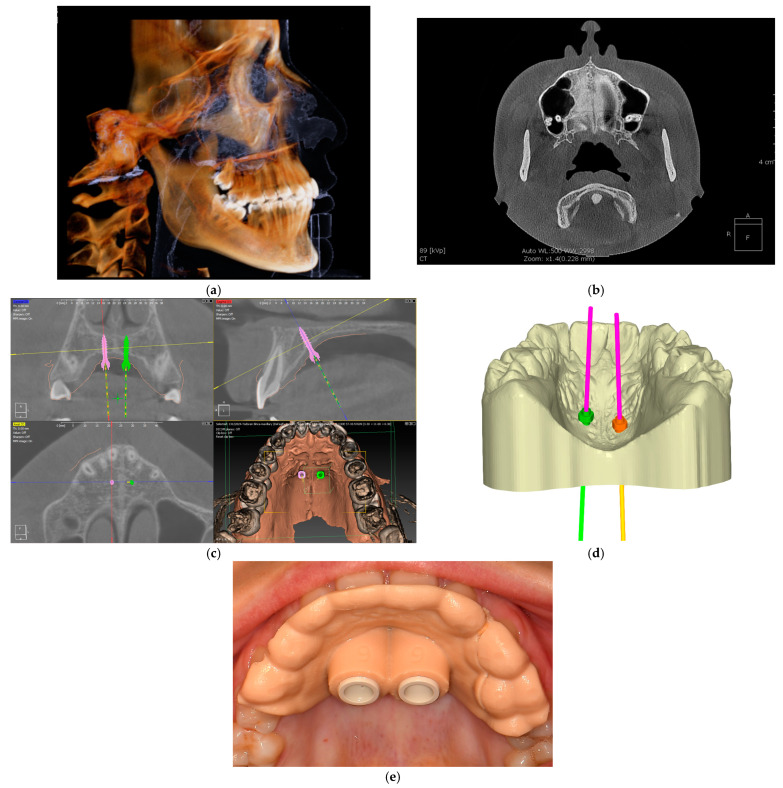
Steps for fabricating 3D surgical guides for orthodontics: (a) 3D image (cone beam computed tomography—CBCT) assessment of the patient 3D reconstruction of the cranium, (b) coronal view of the midpalatal suture, (c) digital planning of the mini-implants placement on the CBCT and (d) virtual model, (e) intraoral verification of the guide.
